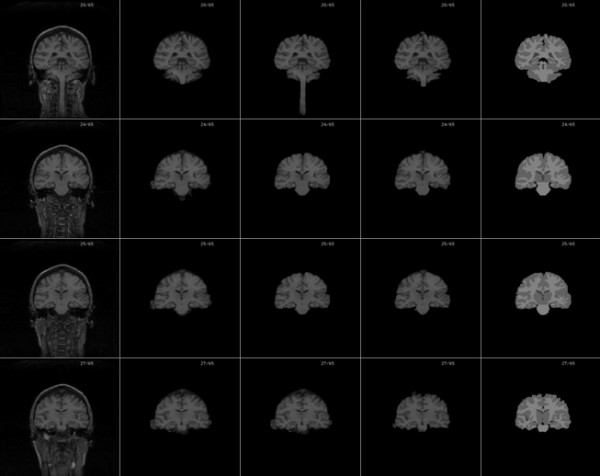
Comparison results of BET, MLS and the proposed method using four slices of normal T1-weighted MR brain images shown in coronal orientation. Columns from left to right: raw image, brain extraction results of BET, MLS, our method and manual extraction result.
